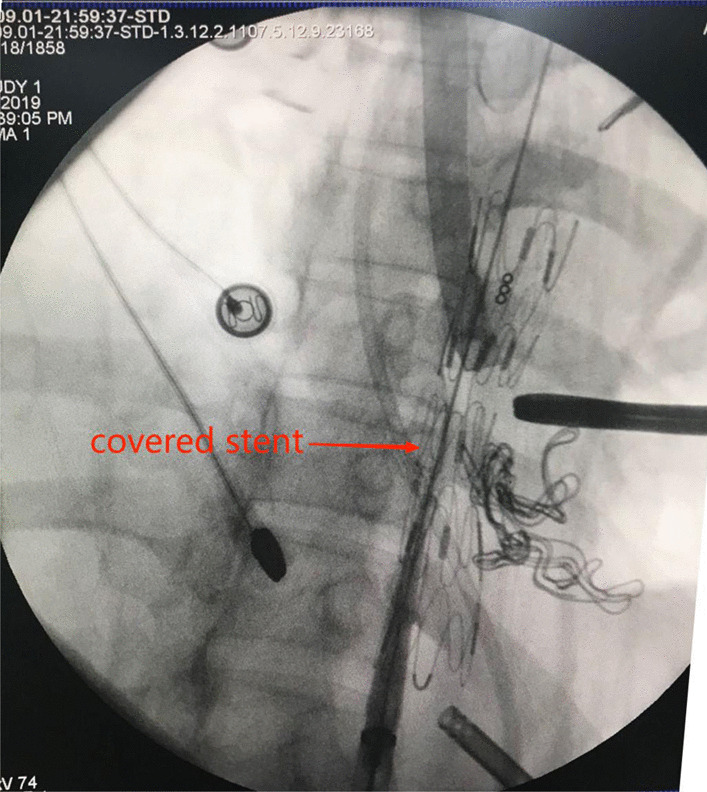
After exploratory thoracotomy and TEVAR (X-ray)
